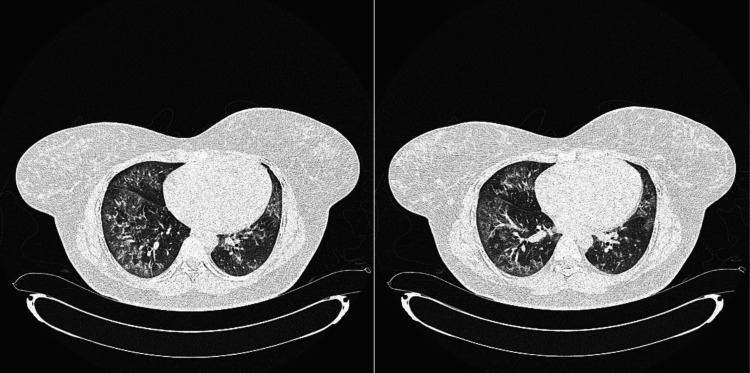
Non-contrast chest CT revealing diffuse ground glass opacities and bronchocentric patchy areas of consolidation
